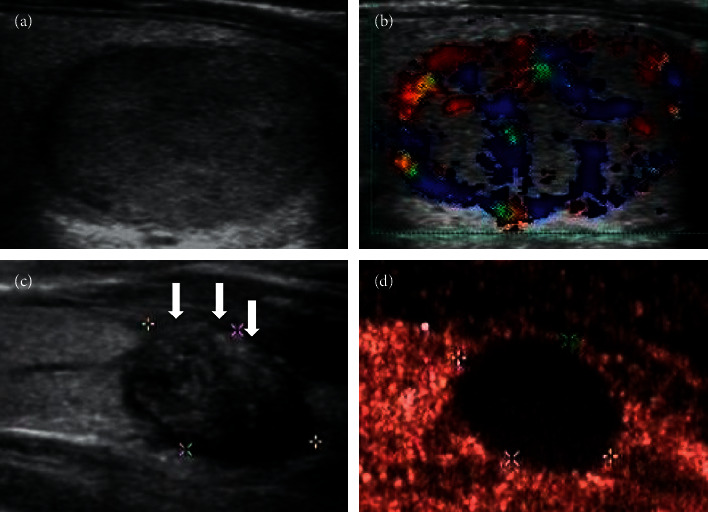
(a, b) A 23-year-old female had an oval solid nodule in the thyroid. The nodule had clear margin and abundant peripheral and internal blood flow signals. The nodule was classified as TI-RADS 3 and considered to be a follicular adenoma. (c) The patient received ultrasound-guided microwave ablation. 1 year after treatment, the nodule became smaller. The anterior capsule of the thyroid was interrupted because of thermal ablation. (d) CEUS showed no enhancement in the nodule suggesting complete necrosis.
